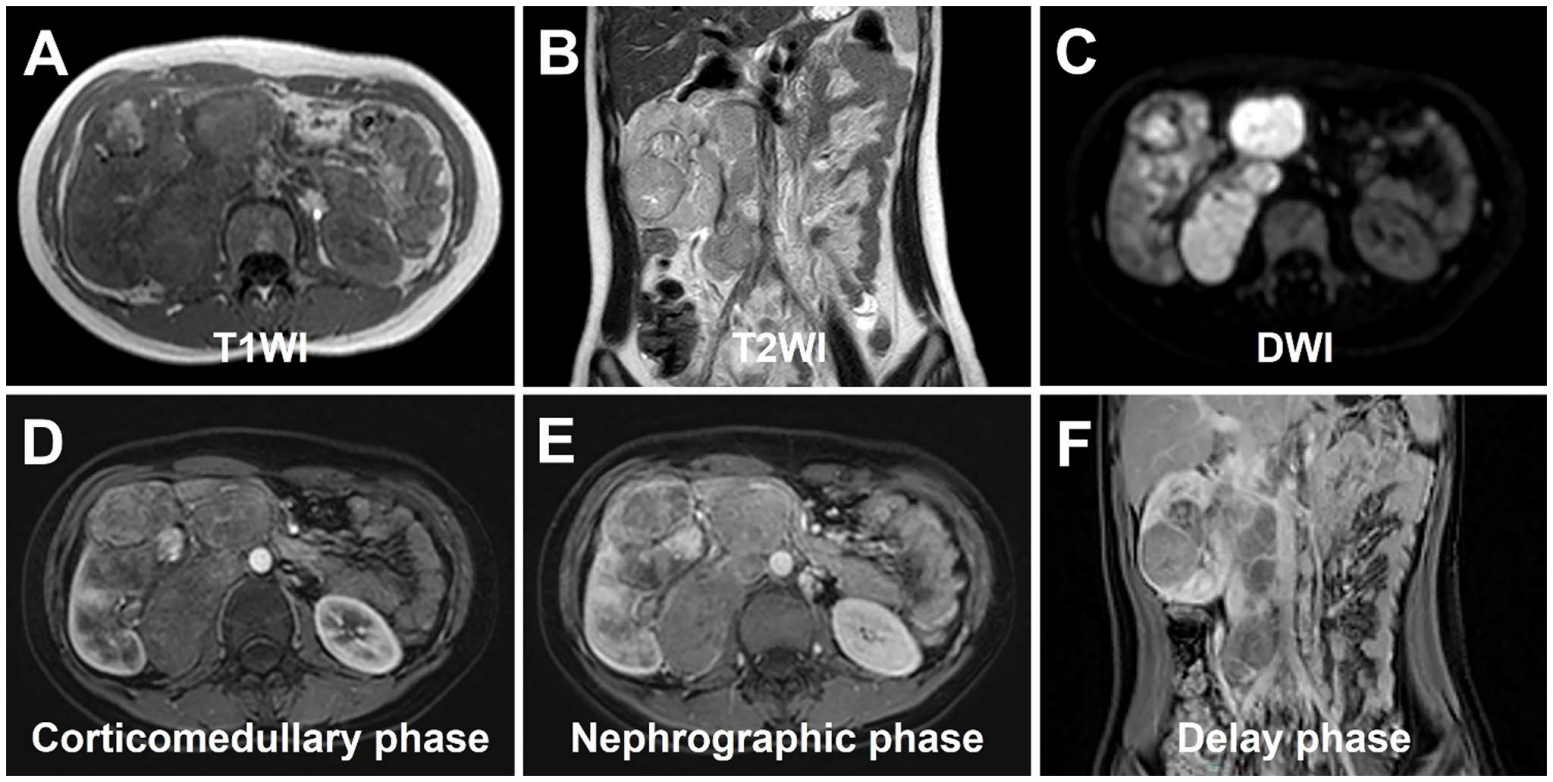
magnetic resonance urography (MRU) of Xp11.2 RCC. (A) T1WI transverse image shows a heterogeneous isosignal lesion. (B) T2WI transverse image shows a heterogeneous slightly low signal lesion. (C) DWI transverse image shows a slightly high signal lesion. (D–F) The degree of enhancement in the three phases of enhancement scan was lower than that in the renal parenchyma, and hemorrhage and necrosis were observed within the internal part of the lesion. Additionally, pseudocoated membrane was observed around the lesion.
